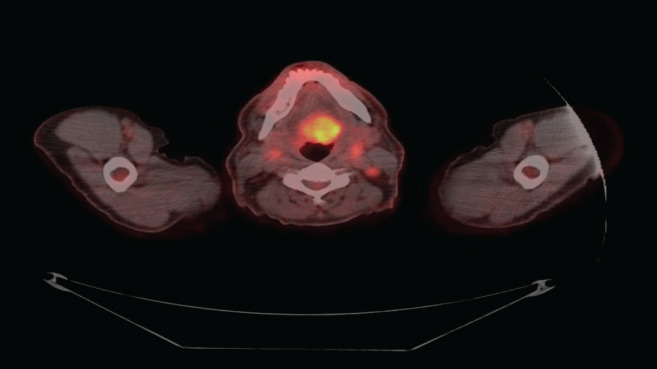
FDG PET increased uptake of FDG in the root of the tongue.
